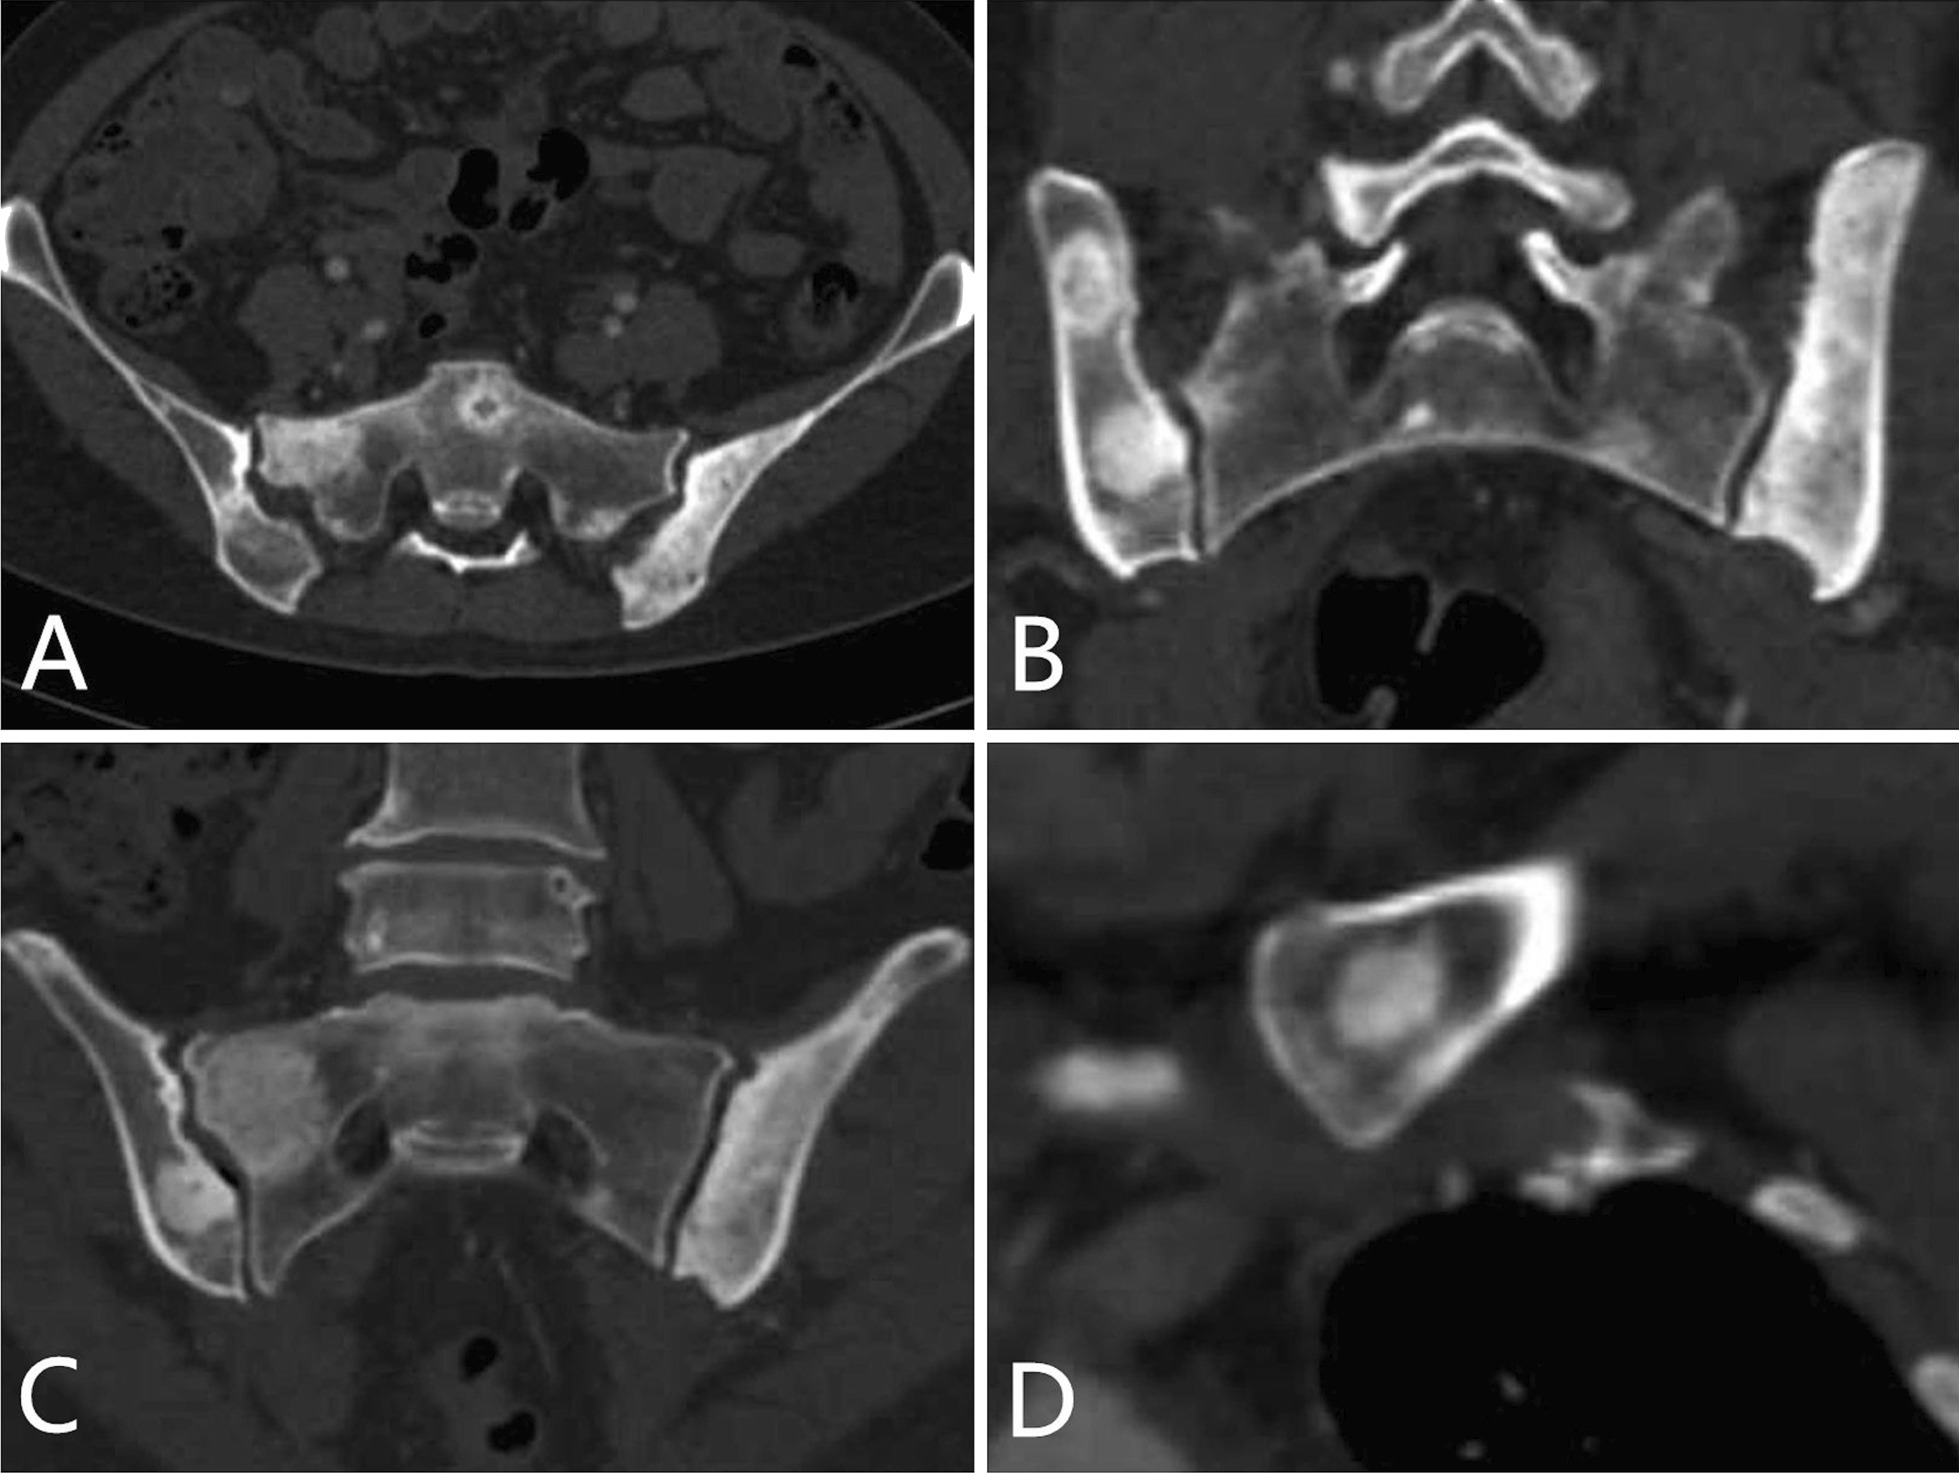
CT images in a 47-year-old woman with pelvis, spine, and clavicles involved. A Axial and B, C coronal reconstruction pelvic CT images show heterogeneous diffuse osteosclerosis filling the left iliac bone, as well as multiple focal osteosclerotic lesions with diverse appearance of nodular and patchy shape in the right iliac bone and the sacrum. D Coronal reconstruction chest CT image shows nodular osteosclerosis in the left proximal clavicle
